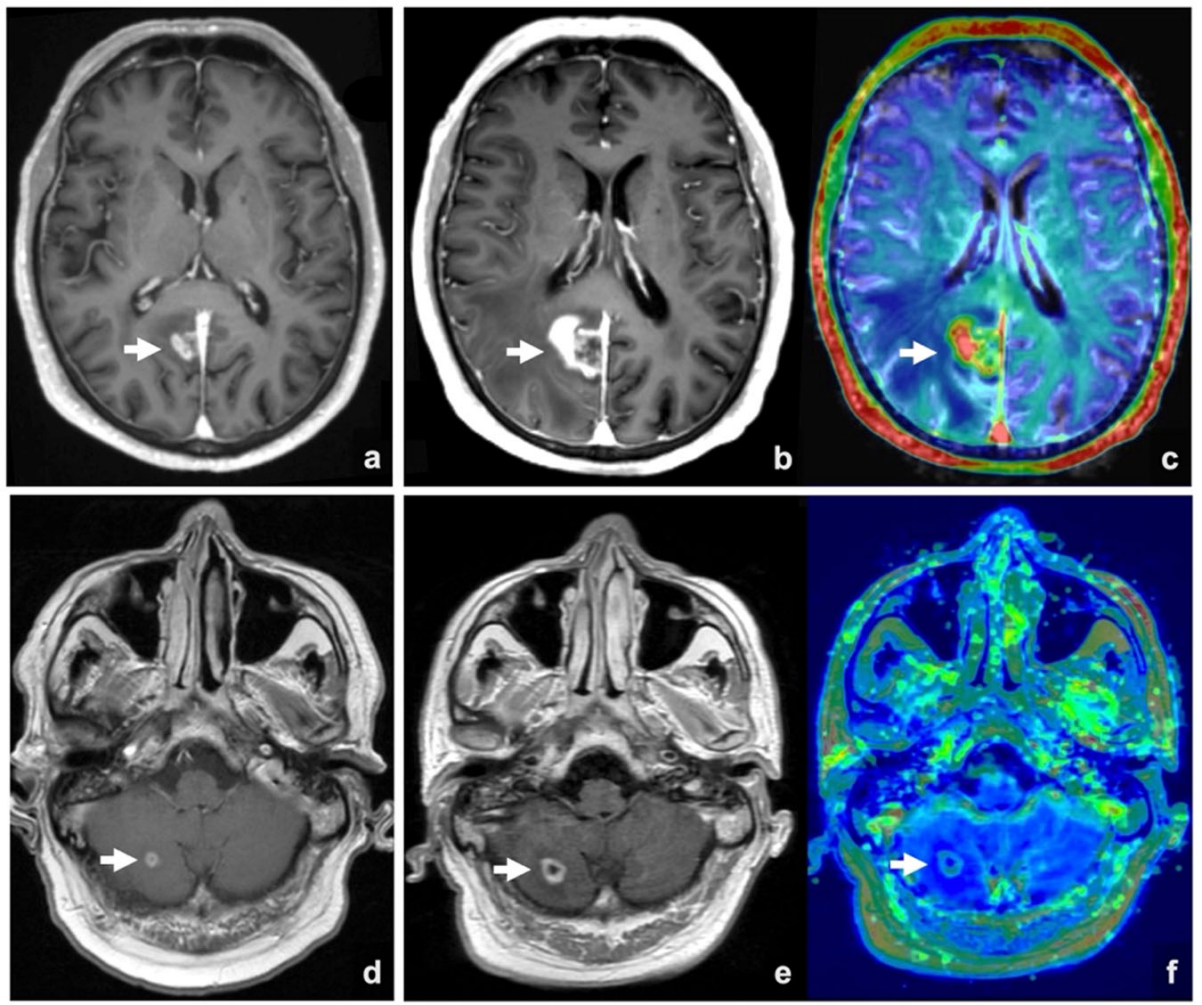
Perfusion MRI in two different patients with brain metastases from NSCLC treated with Pembrolizumab. The first patient was treated without concomitant radiotherapy, while the second also received SRS. In the first patient (a–c), contrast-enhanced T1w brain MRI (a) shows a right occipital lesion (arrow in a). One year later (b), the lesion has increased in size, and perfusion MRI (c) shows a pathological increase in CBV, indicating disease progression. In a second patient (d–f), brain MRI (d) shows a right cerebellar lesion (arrow in d). Five months later (e), the lesion has increased in size; however, perfusion MRI (f) shows no significant increase in CBV, findings that are consistent with post-treatment changes
